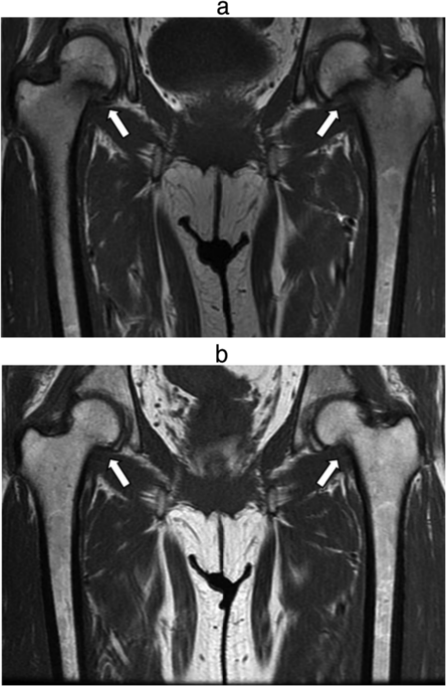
MRI findings of incomplete bilateral transcervical fractures (Arrows) before (a) and after (b) the treatment
